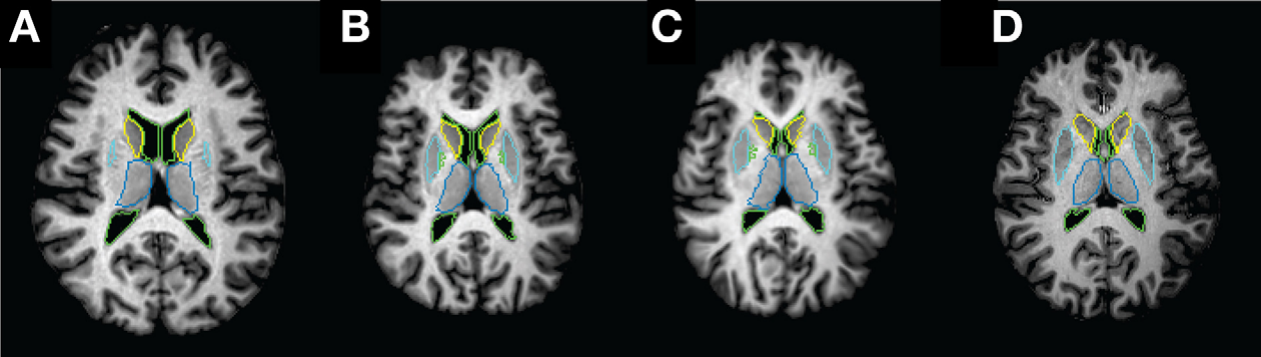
Example axial slices of atlas and subject T1 weighted MR image and the boundaries of their ROI labels at each registration step. (A) Original atlas image and atlas ROIs; (B) atlas image and ROIs after linear AIR transformation; (C) atlas image and ROIs after AIR and LDDMM transformations; (D) subject image and subject ROIs.
